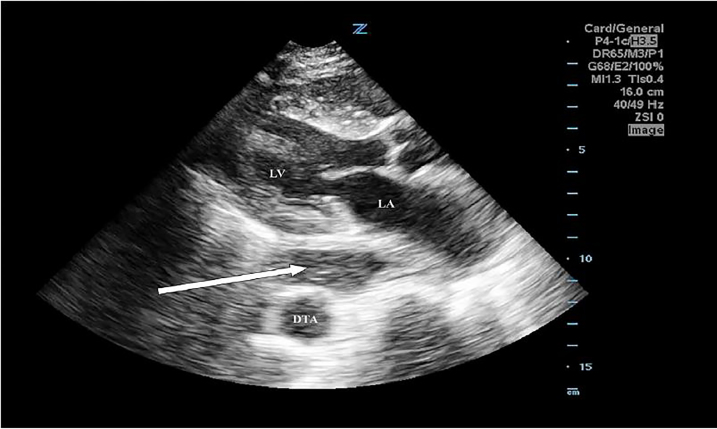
Parasternal long-axis window with anechoic mass (arrow) with microbubbles posterior to the left atrium (LA) and left ventricle (LV) but anterior to the descending thoracic aorta (DTA). Despite mimicking life-threatening aortic pathology, this is a fluid-filled hiatal hernia.
